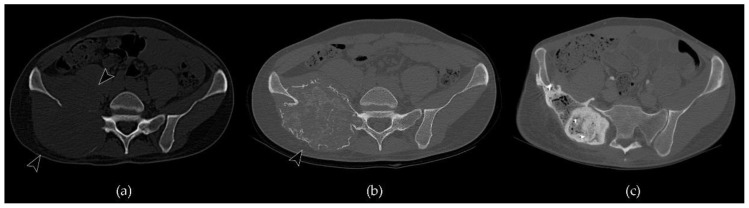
Axial CT scan of a case of GCTB of the sacrum and right iliac wing treated with denosumab and then subjected to surgical osteotomy: (a) a pre-treatment image shows an extensive osteolytic lesion, which extends to the adjacent soft tissues, involving the gluteal area and the pelvis (arrowheads); (b) a post-denosumab image demonstrates peripherical deposition of bone matrix with neocortex formation and intralesional osteosclerosis (arrowhead). A modest size reduction of the lesion can also be noted in relation to the shrinkage effect; (c) post-surgical evaluation highlights the outcomes of osteotomy in the right iliac wing filled with cement and two K-wires.
